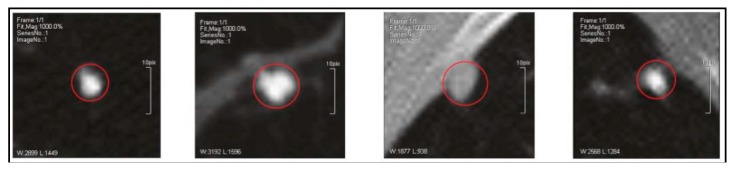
Demonstration of four types of lung nodule CT image, shown from left to right, W, V, J, and P, respectively [12]. Red circles denote the locations of the nodule.
